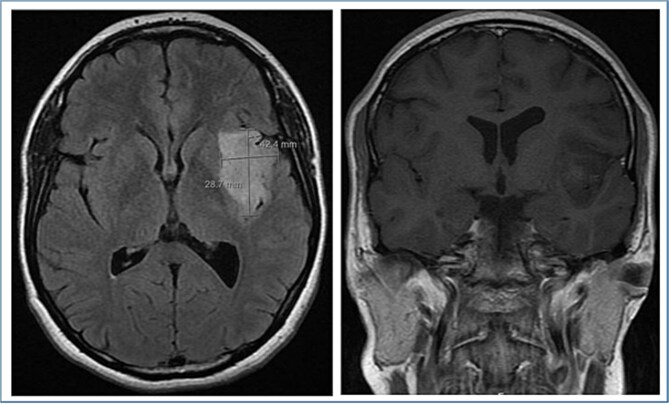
Preoperative brain MRI demonstrates a large, well-defined, expansile intra-axial mass lesion centered in the left insular region with cortical and subcortical involvement.
